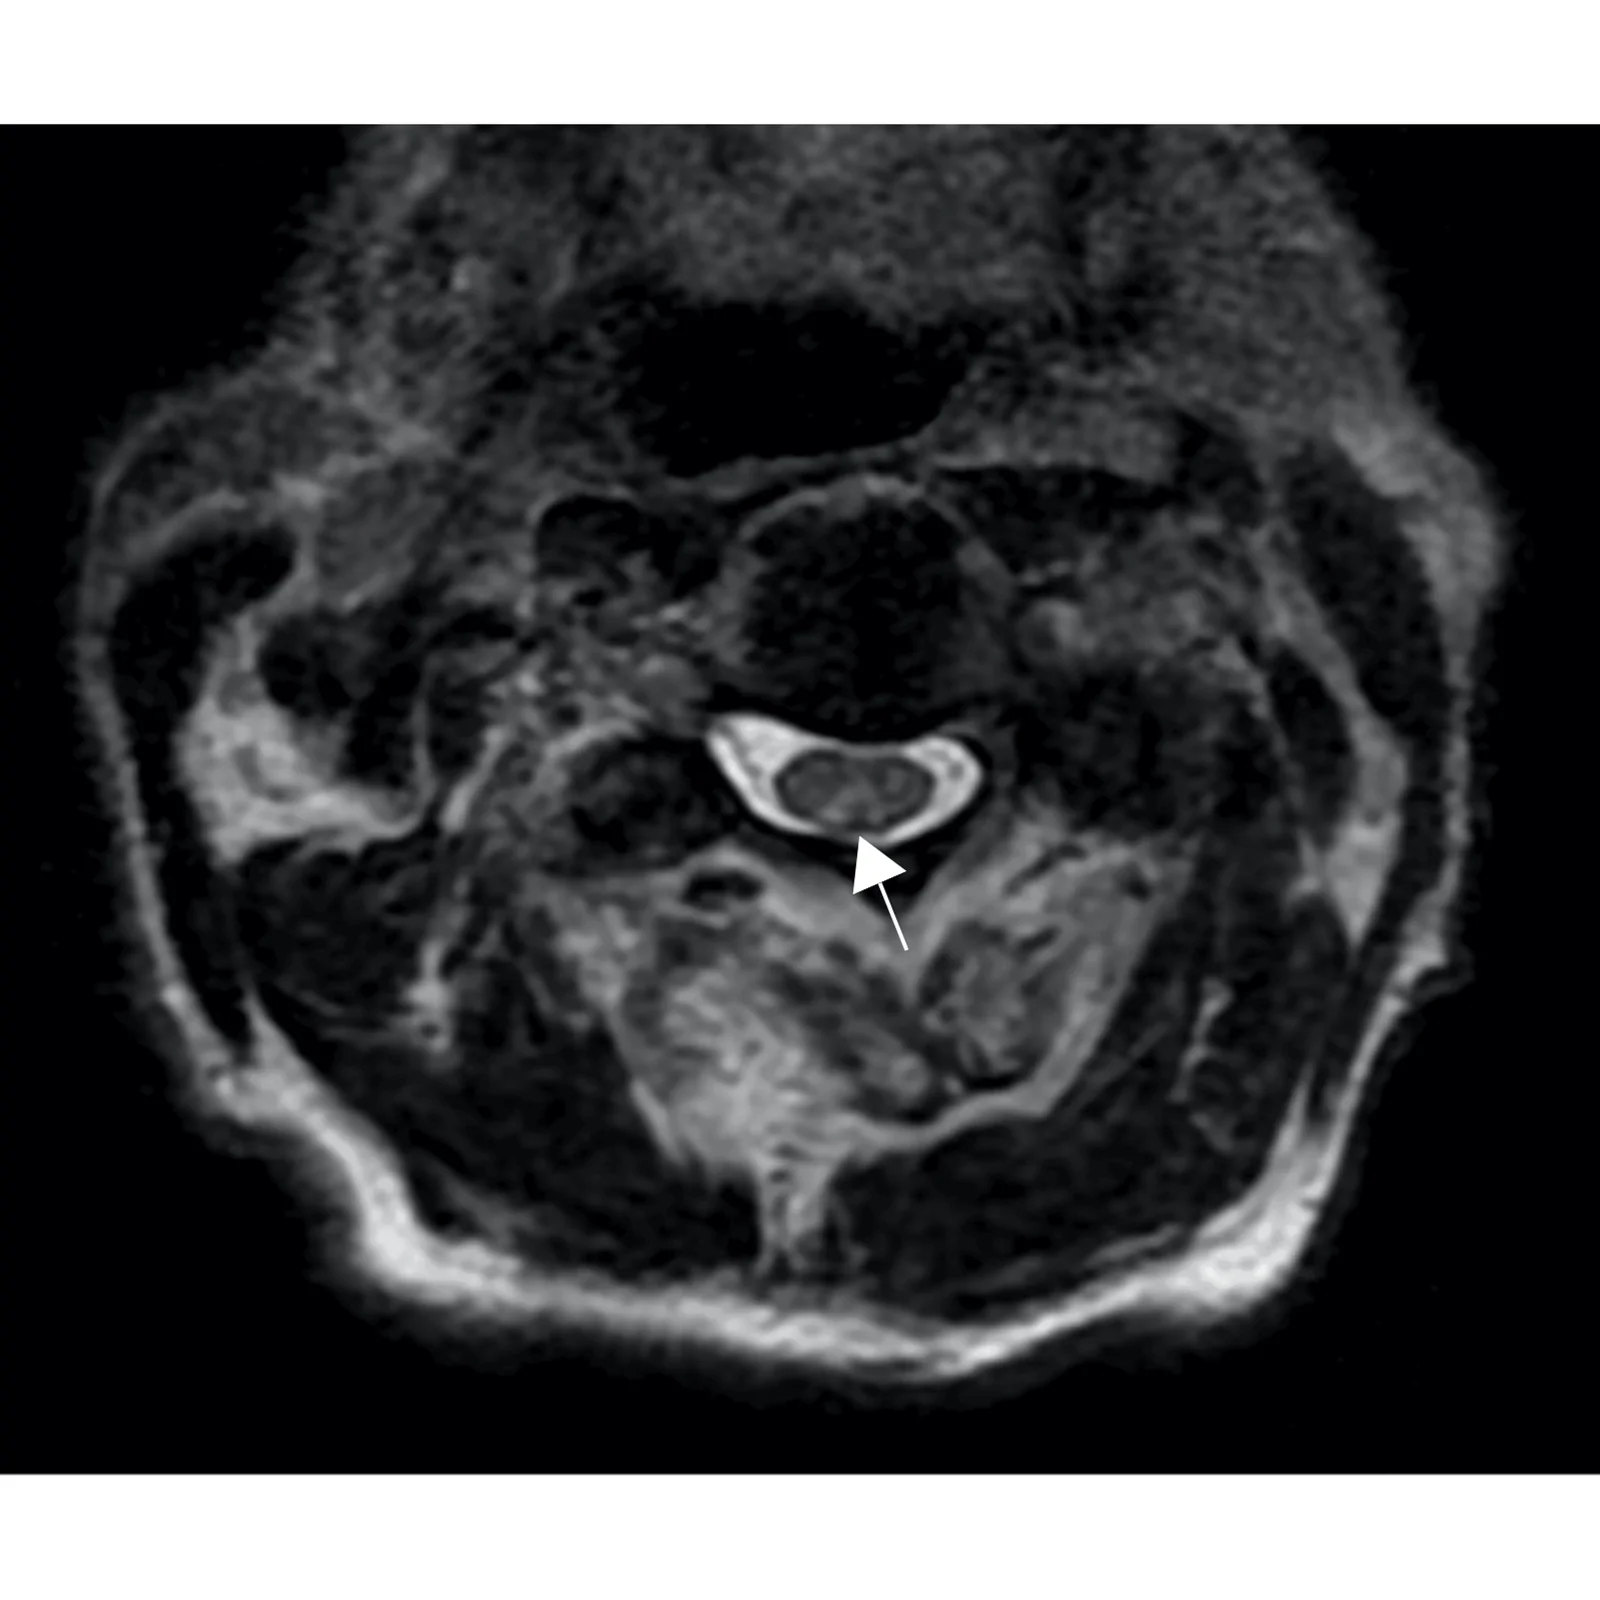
Magnetic resonance imaging (MRI) of the cervical spine Axial view-T2 sequence revealed a bilaterally symmetric hyperintensity in the cervical dorsal columns, forming the characteristic "inverted V" consistent with subacute combined degeneration.
